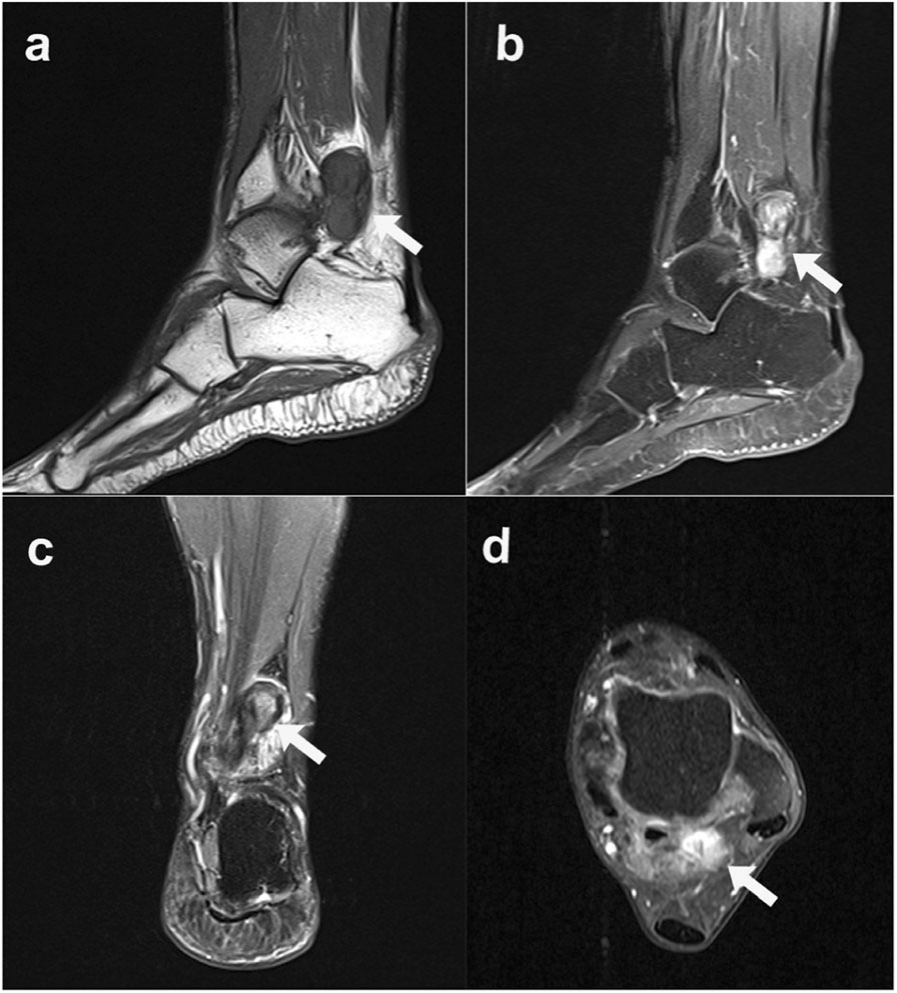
Magnetic resonance images of the patient’s left ankle. a Sagittal T1-weighted turbo spin echo (TSE) image. b Sagittal T1-weighted TSE contrast-enhanced image with Ga-DTPA fat -suppressed. c Coronal proton density (PD)-weighted TSE fat-suppressed image. d Transverse T1-weighted TSE contrast-enhanced image with Ga-DTPA fat-suppressed. A well-enhanced mass was identified beside the flexor hallucis longus muscle at the level of the ankle joint (arrows). Considering that the patient was diagnosed with melanoma, these appearances were in favor of metastases from melanoma according to a radiologist. However, the differential diagnosis was pigmented villous nodular synovitis
